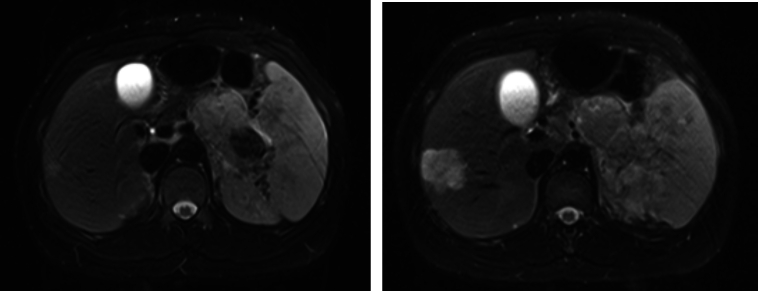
Axial view from a T2-weighted MRI revealing a suspected tumor in the pancreatic body and tail, with liver and splenic metastases.
